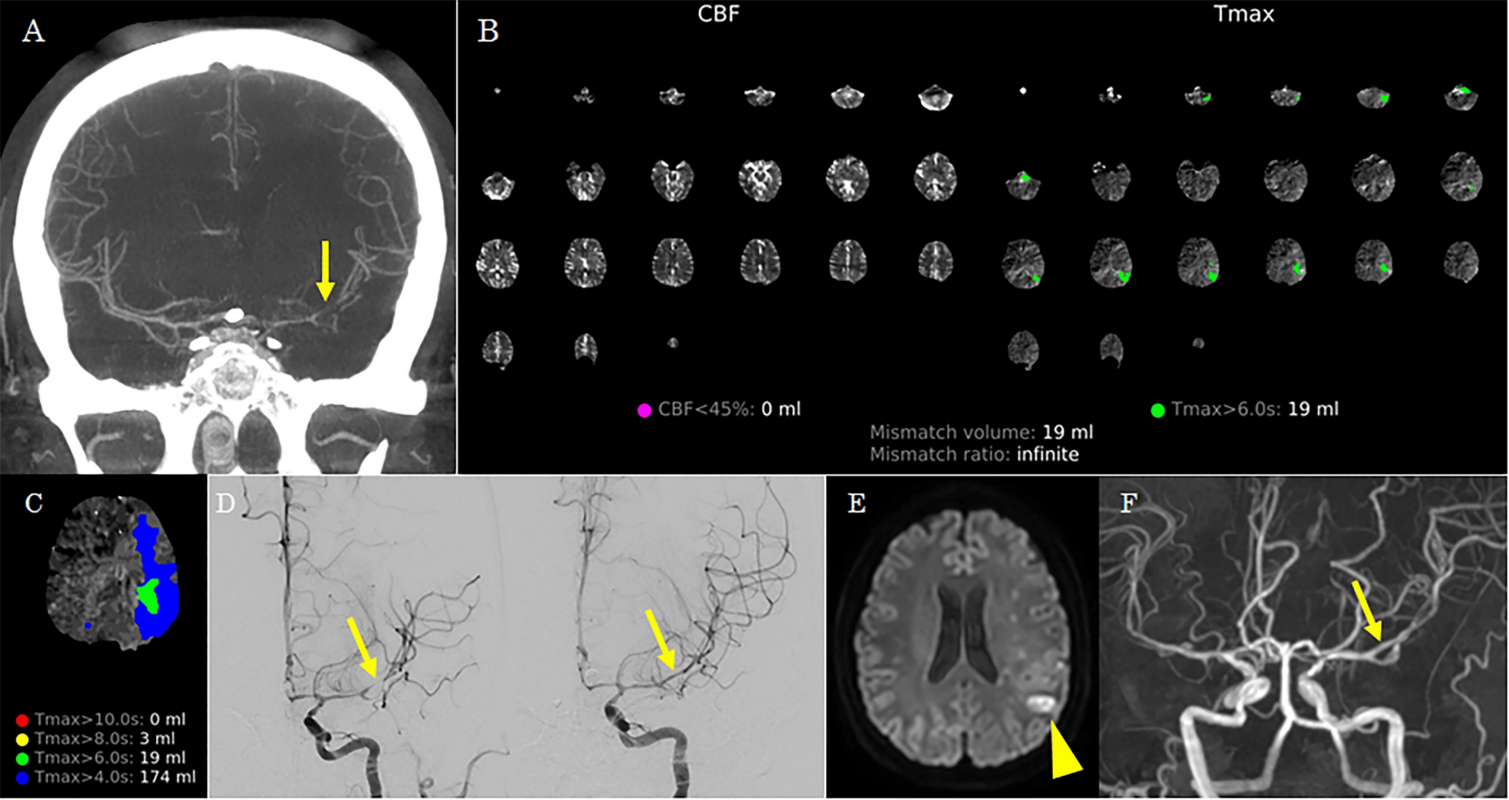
Case 1. (A) CT angiography-like images from the RAPID ANGIO show narrowing of the left middle cerebral artery (arrow). (B) Automated perfusion maps from RAPID ANGIO demonstrate no infarct core (defined as cerebral blood flow <45%) but reveal significant hypoperfused tissue with a time-to-maximum (Tmax) >6 seconds in the left cerebral hemisphere. (C) Detailed Tmax map from the RAPID ANGIO suggests mildly delayed perfusion (Tmax >4 seconds) in the left cerebral hemisphere. (D) Left carotid angiography showing improvement of severe vasospasm in the left middle cerebral artery after intra-arterial administration of fasudil hydrochloride and percutaneous transluminal angioplasty (arrow). (E) Post-procedural diffusion-weighted MRI revealing a small infarction corresponding to the hypoperfused region identified on RAPID ANGIO (arrowhead). (F) Post-procedural MR angiography shows no residual narrowing (arrow).
